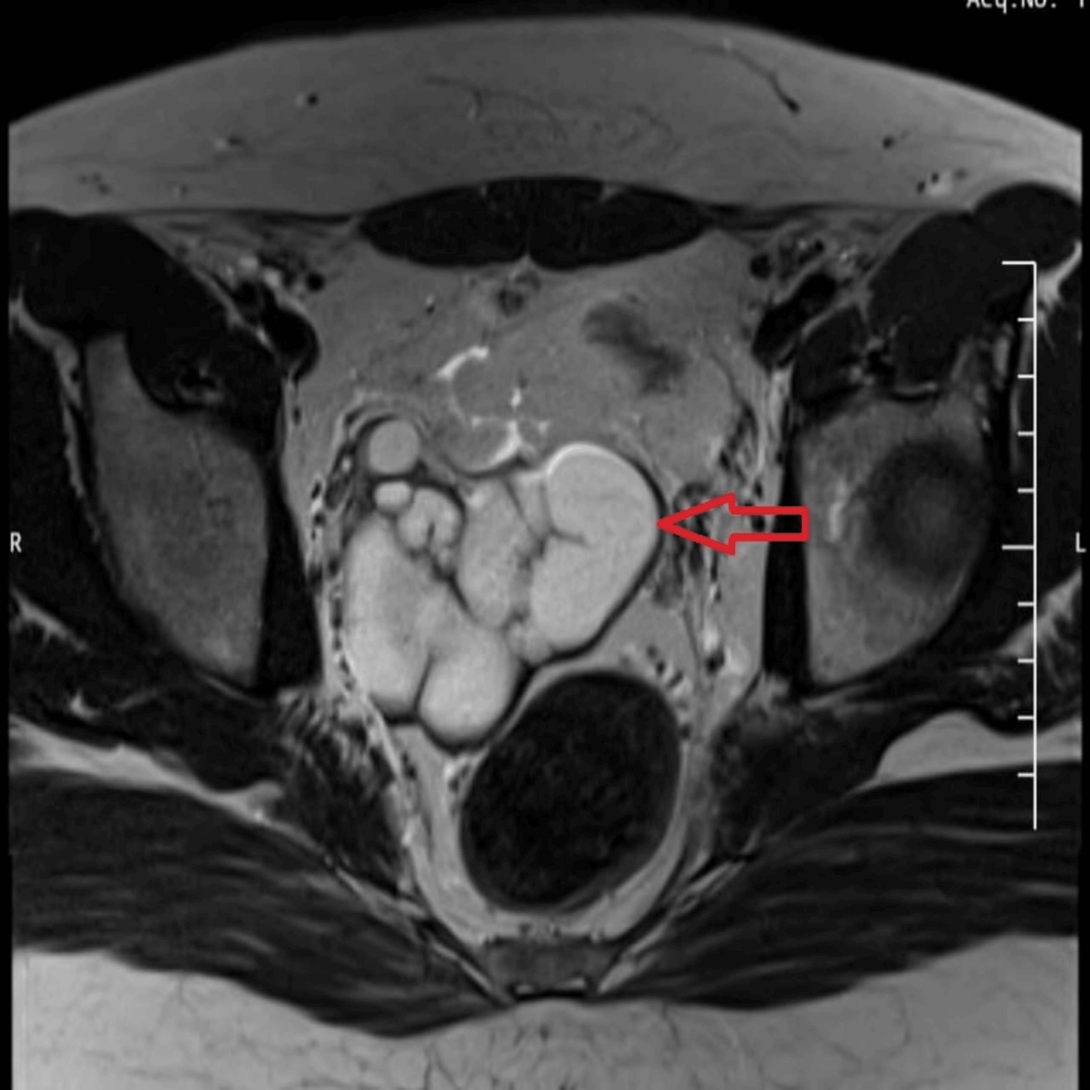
Axial T2-weighted MRI pelvis image reveals a hyperintense, tubular seminal vesicle cyst with incomplete internal septations adjacent to the prostate (arrow). MRI, Magnetic resonance imaging
